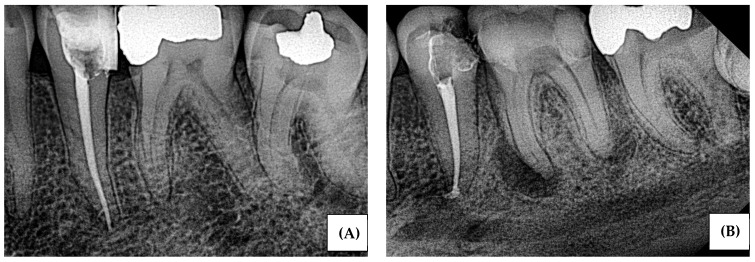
Example periapical radiographs: (A) abnormal image with periapical radiolucency; (B) normal image with intact lamina dura and no periapical radiolucency.
